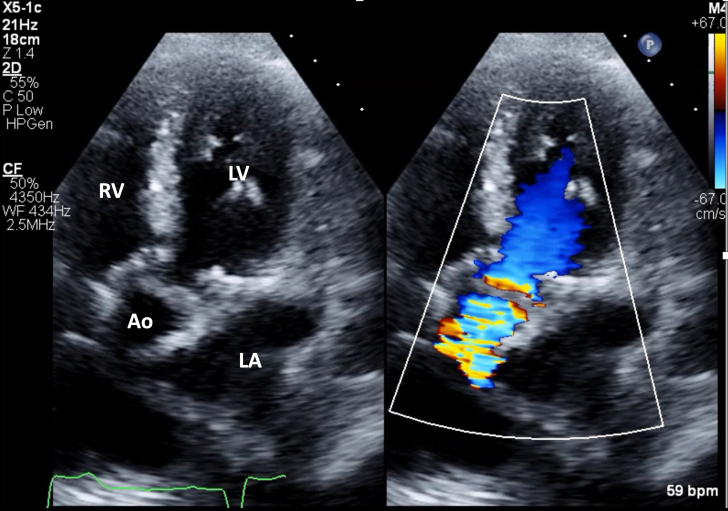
Apical 5-Chamber View Evaluation for Aortic Stenosis With and Without Color Doppler Apical 5-chamber view with color Doppler of the aortic valve to evaluate for the stenotic mosaic color flow pattern caused by an outflow obstruction and also for aortic regurgitation. Abbreviations as in Figure 2.
